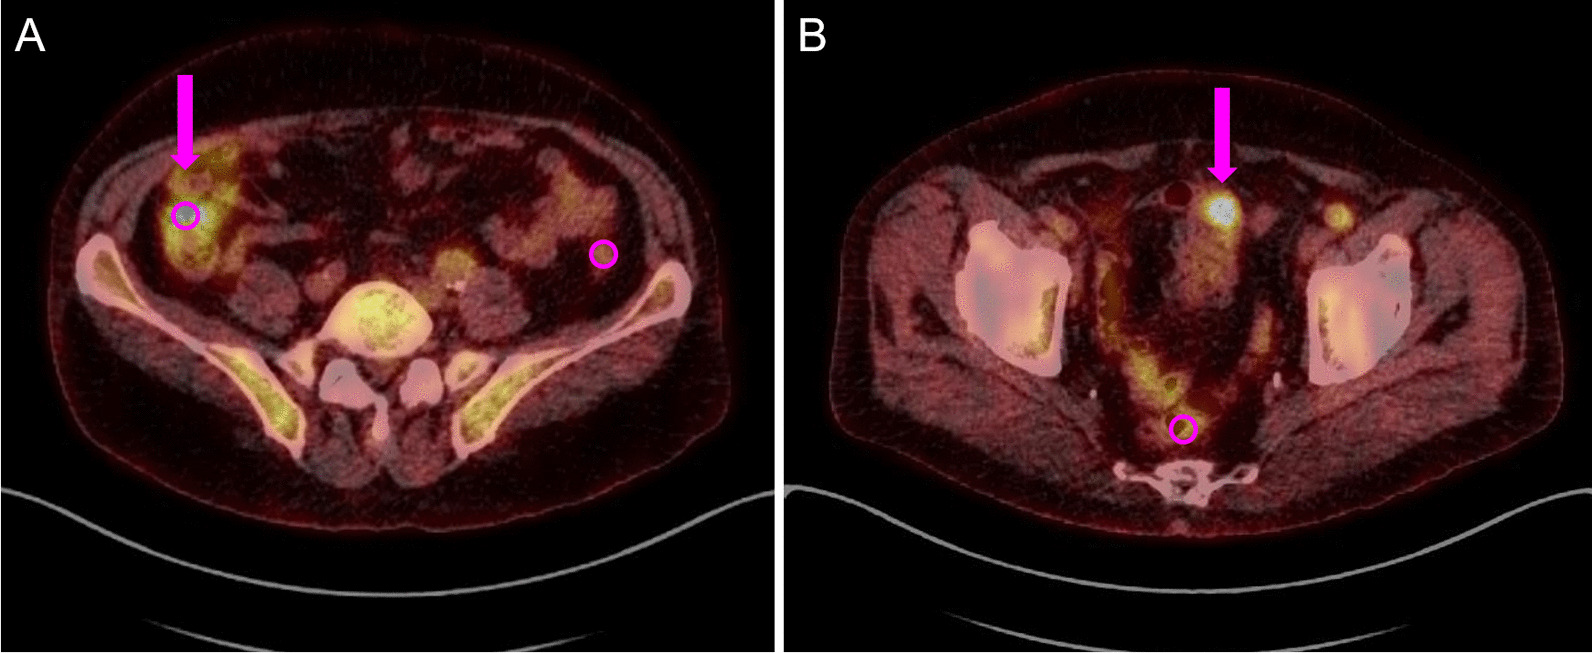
Exemplary depiction of FDG uptake in the bowel of two patients, representing quantitative and subjective assessment. A Uptake in the ascending colon (arrow); intensity: moderate, focality: diffuse, probability of being pathologic: probable physiologic, theoretical endoscopy recommendation: no. B Uptake in the sigmoid colon (arrow); intensity: high, focality: focal, probability of being pathologic: probable pathologic, theoretical endoscopy recommendation: yes. Standard sized spherical measurement regions (VOI) of SUV_mean_ shown as circles with a diameter of 1.0 cm in ascending colon, descending colon (both A) and rectum (B)
